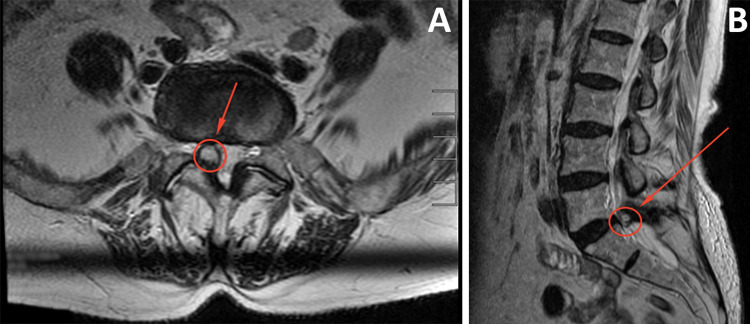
(A) Preoperative axial view of the facet cyst indicated by the circle and arrow in T2 as seen on magnetic resonance imaging. (B) Sagittal view of the cyst. The patient’s significant radicular symptoms can be correlated with the stenosis caused by the cyst.
